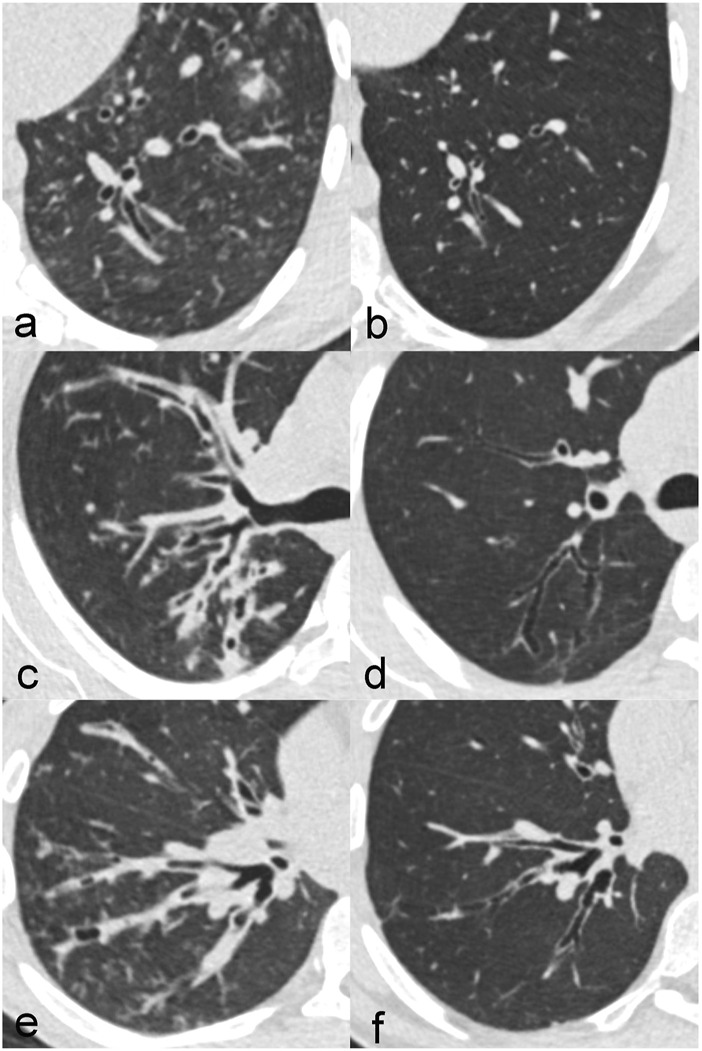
Improvement of CT changes in three different patients with CF between at baseline before treatment (A,C,E) and at follow-up after treatment initiation with elexacaftor-tezacaftor-ivacaftor (B,D,F). Patient 1 (A,B) is a 20-year old male with impressive regression of bronchiectasis. Patient 2 (C,D) is a 24-year old female in which peribronchial thickening clearly decreased. Patient 3 (E,F) is a 20-year old male with a reduction of mucus plugging.
